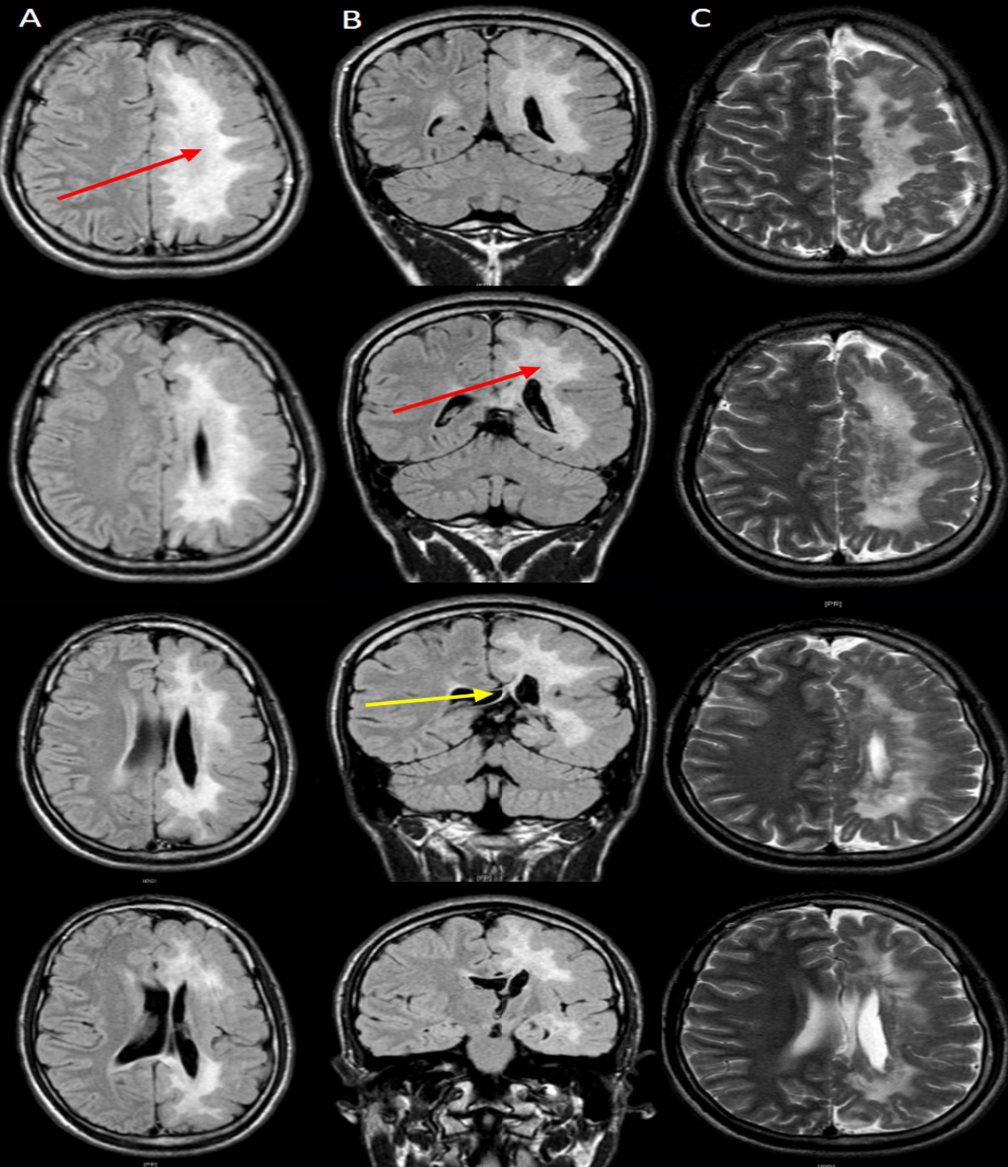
T1 and T2-weighted MRIs in columns A, B, and C, respectively, show left cerebral hemisphere diffuse white matter loss (red arrows) and midline shift (yellow arrow) due to corpus callosum volume loss.
